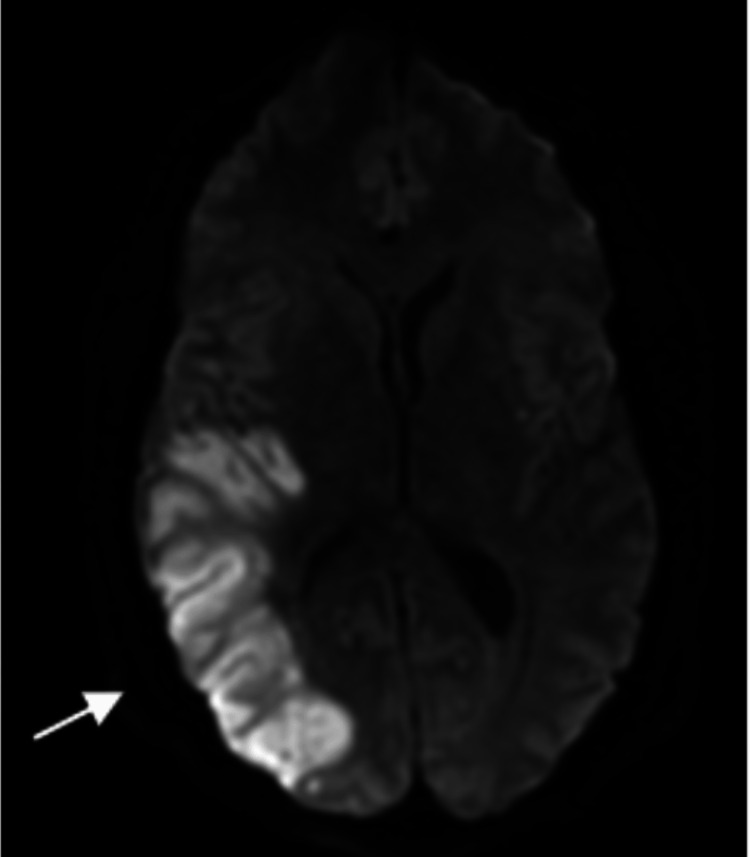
MRI brain diffusion-weighted imaging series showing restricted diffusion in temporal, parietal, occipital regions
